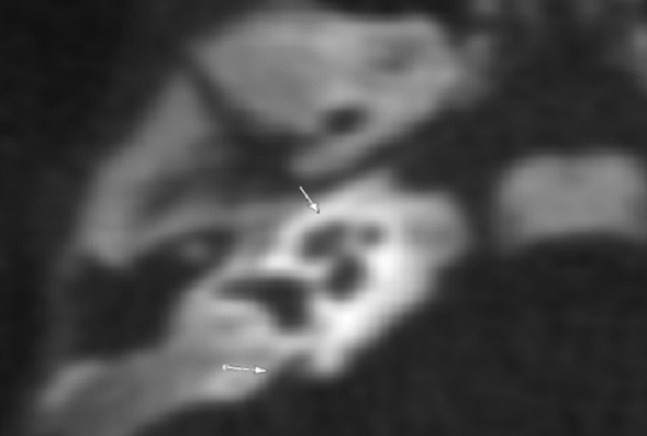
CT scan of temporal bone shows: Upper arrow: incomplete number of turns. Lower arrow: Widened vestibular aqueducts
